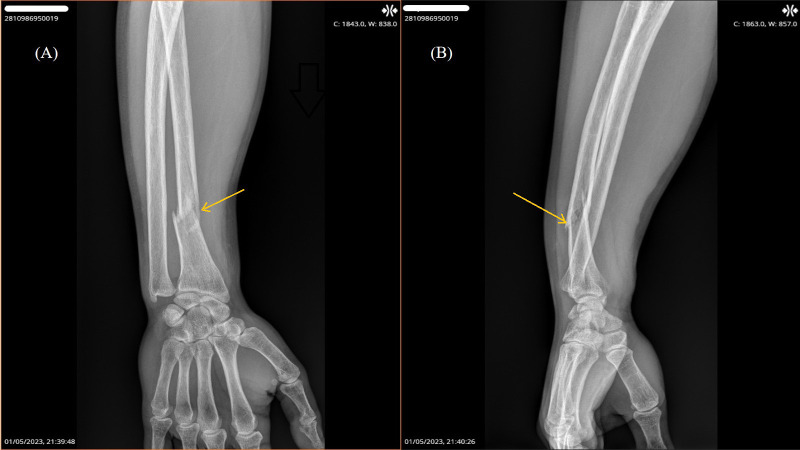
X-ray of the right radius showing a pathological fracture: (A) anteroposterior view; (B) lateral view.
